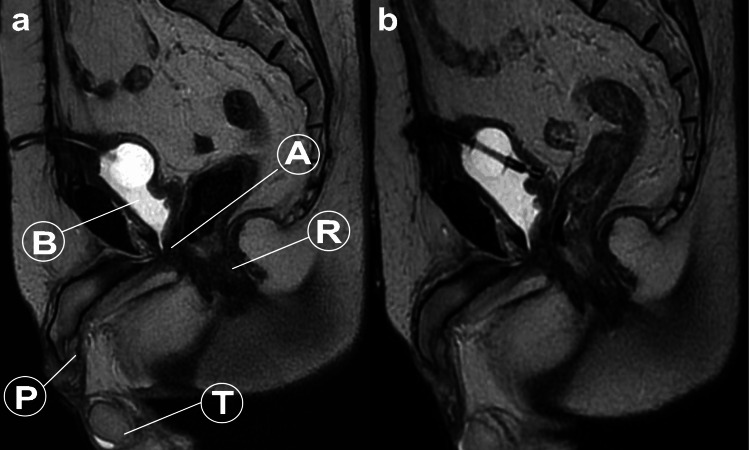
MRI Sagittal Images Before and After Treatment B: Bladder, P: Penis, T: Testes, R: Rectum, A: Anastomosis
